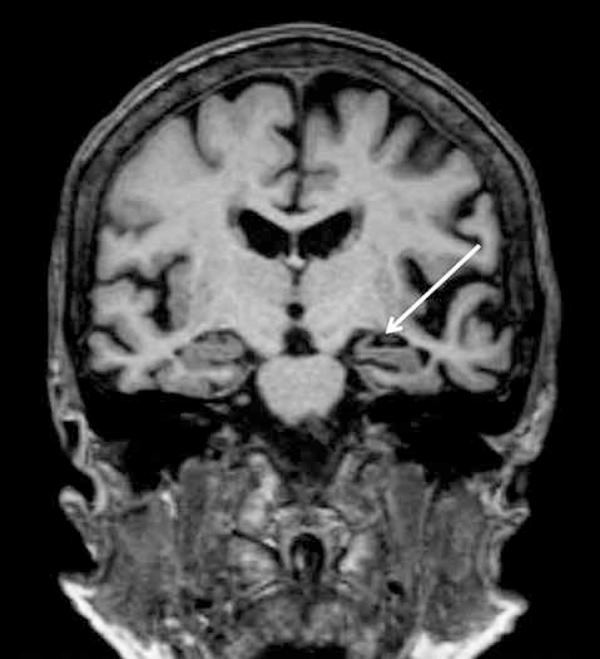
Coronal FLAIR brain MRI image. Image reveals bilateral hippocampal
